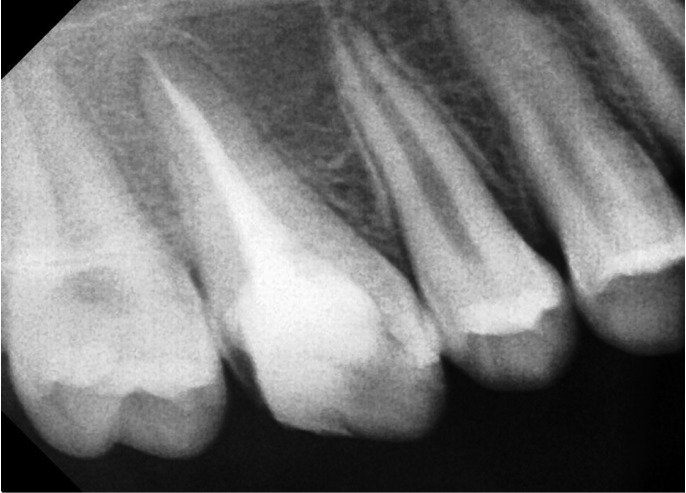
Radiographic view showing 2 year follow up of obturated maxillary first molar.
